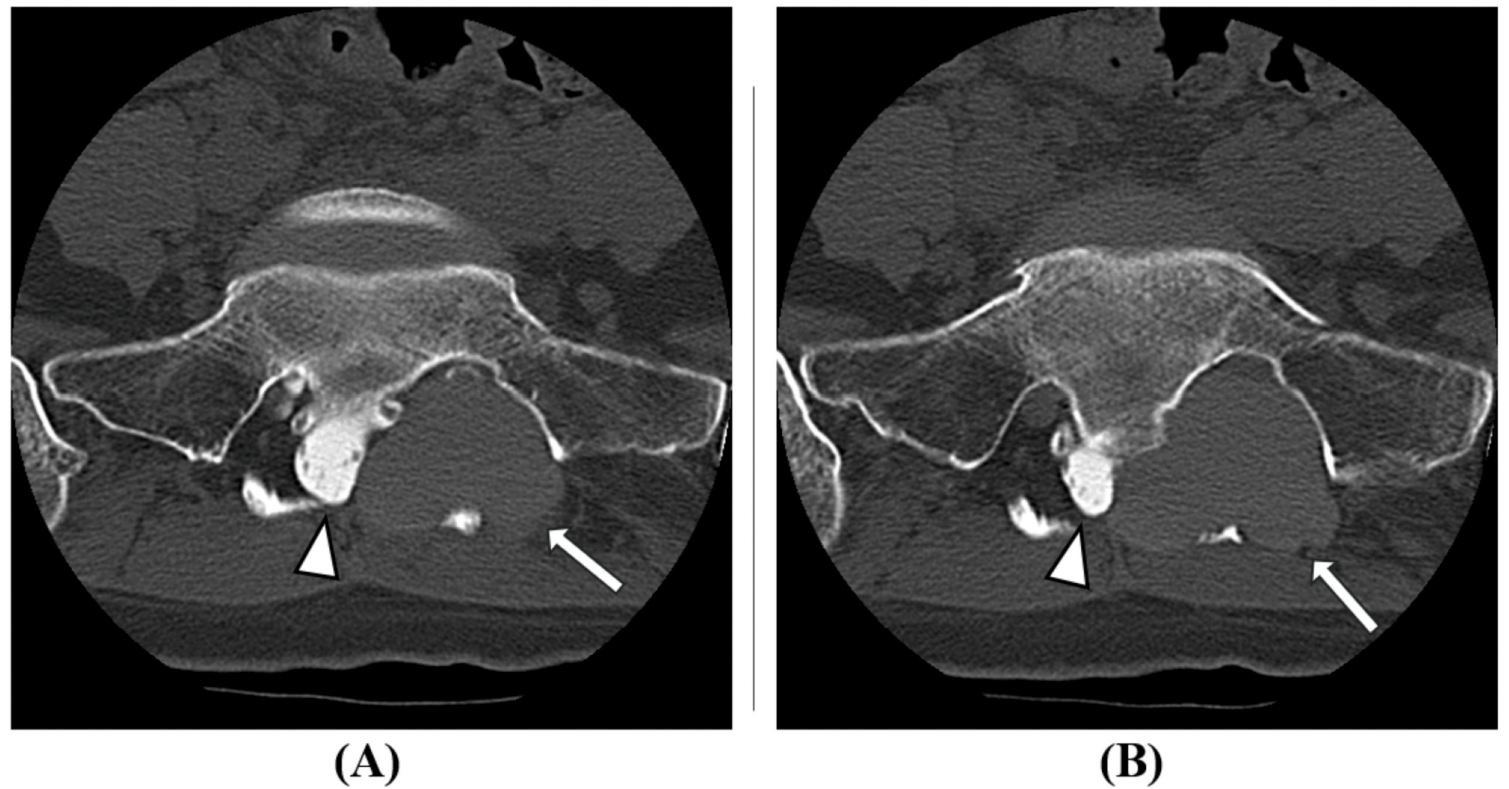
Post-myelography CT axial images (A) S2 level, (B) S3 level Good contrast enhancement is obtained within the spinal canal (arrowheads in A, B), but there is minimal inflow of contrast medium into the cyst (arrows in A, B). This suggested poor communication between the cyst and the subarachnoid space.
